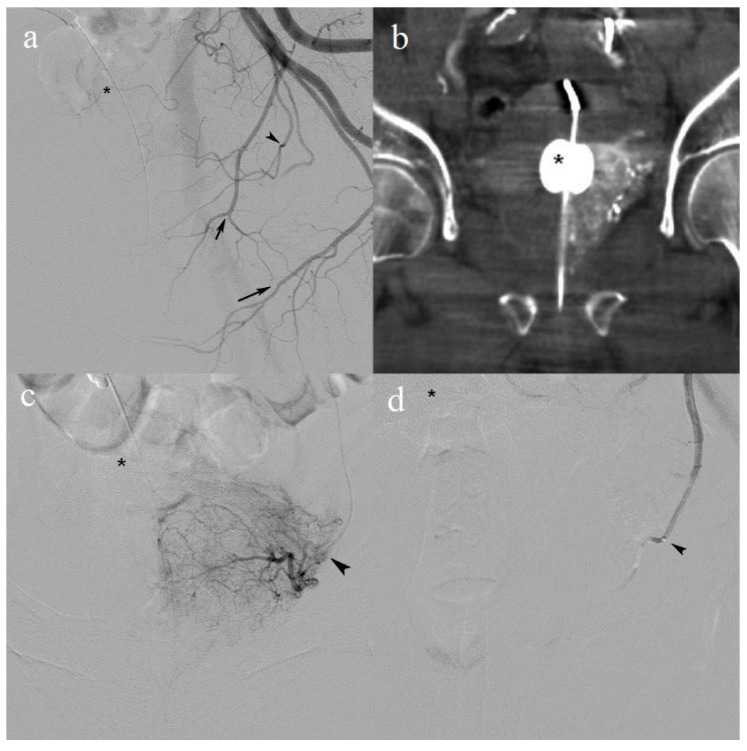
(a) Selective digital subtraction angiography (DSA) in the left anterior oblique position with the catheter tip in the left internal iliac artery (not visible). The internal pudendal artery (long arrow) and the characteristic division of obturator artery (short arrow). The prostate artery (arrowhead) protrudes beneath the Foley balloon (*) catheter; (b) coronal cone-beam computed tomography with contrast enhancement in the left lobe confirming correct catheter placement; (c) frontal DSA before embolization with injection on the microcatheter in the prostate artery (arrowhead); (d) frontal DSA with the microcatheter in the same position (arrowhead) and the angiographic endpoint after embolization.
